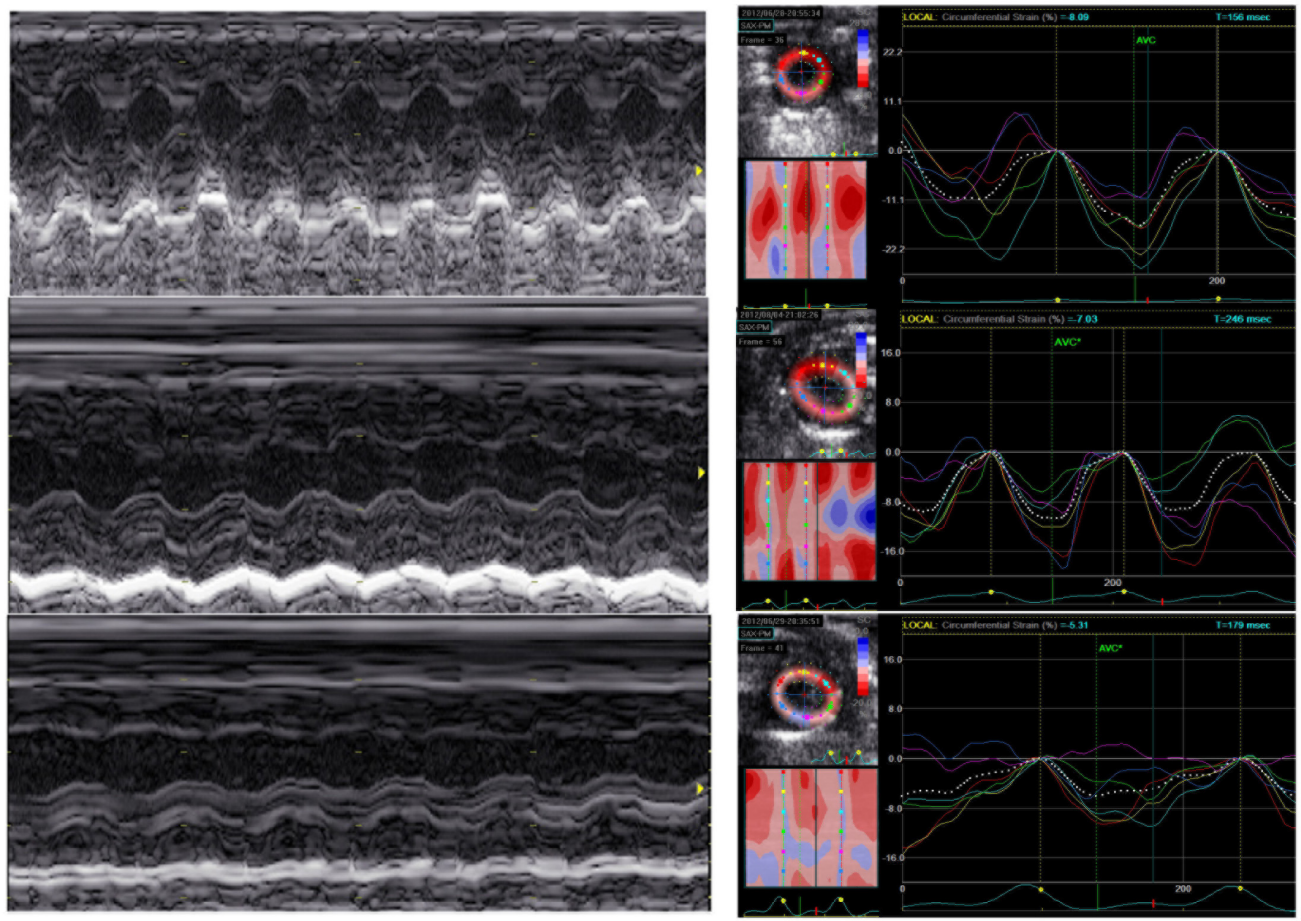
Examples of left ventricular myocardial function assessments by conventional echocardiography (left) and strain echocardiography (right) at baseline (upper plane), after 10 mg/kg LPS challenge (middle plane), and 20 mg/kg LPS administration (lower plane), respectively.
